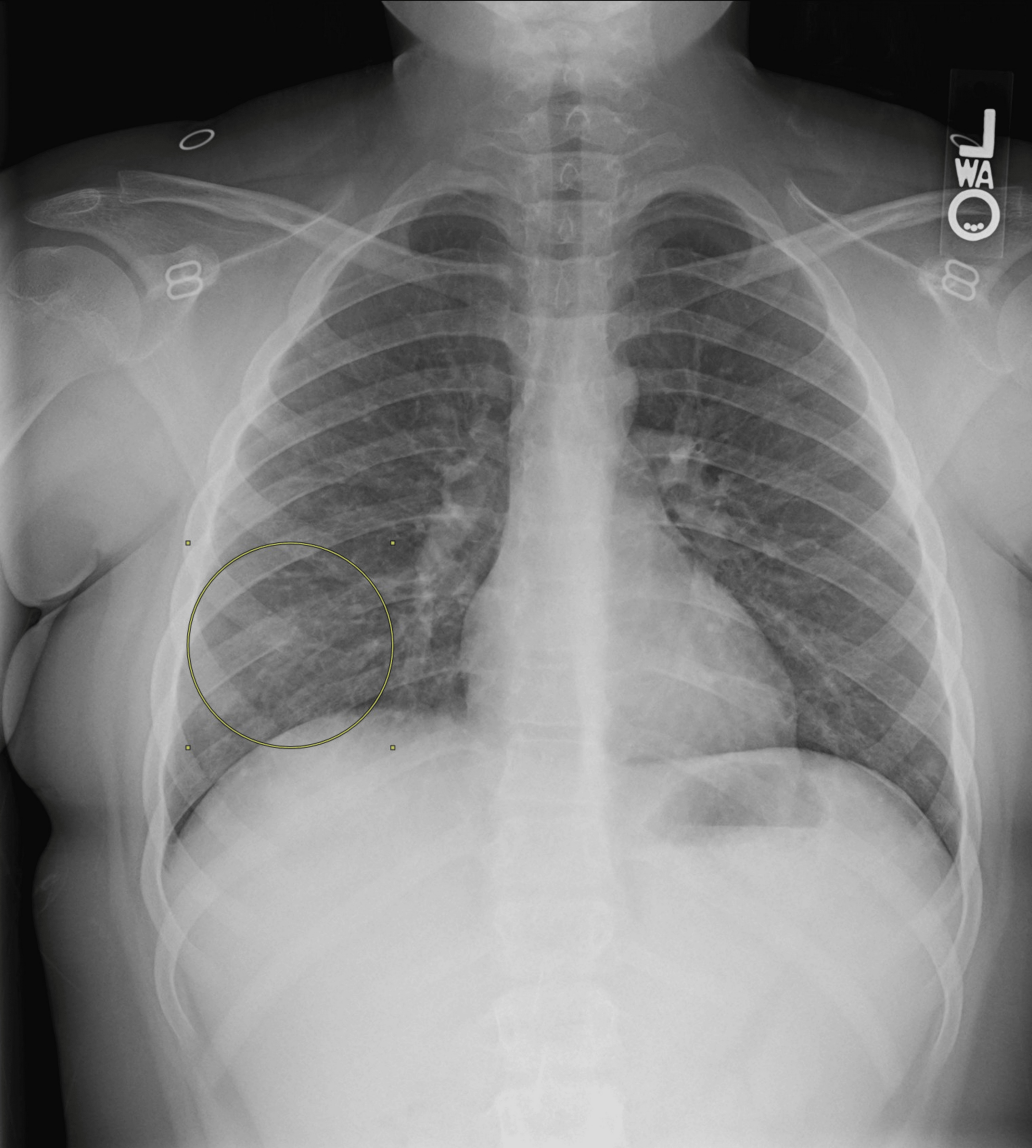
Initial chest X-ray during urgent care evaluation
